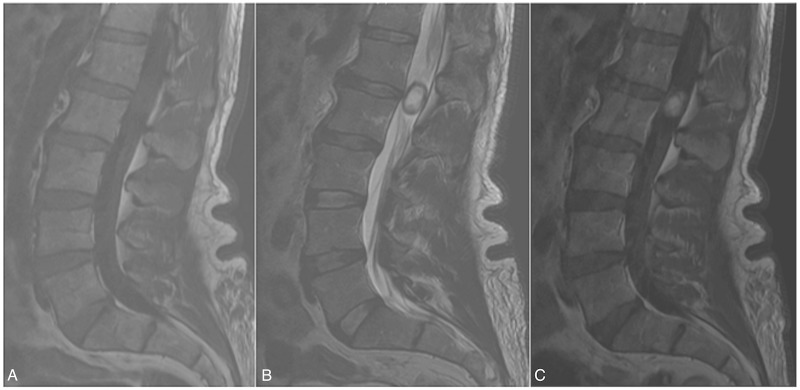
Magnetic Resonance Imaging (MRI) of the Lumbar Spine Magnetic Resonance Imaging without and without contrast a. T1-weighted imaging (T1WI) demonstrating a hypo-/isointense lesion at the L1-2 disc space which is
